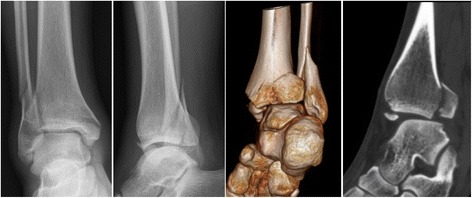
The 55-year-old male patient treated using indirect reduction technique. Preoperative radiographs and CT images showed a displaced PMF
